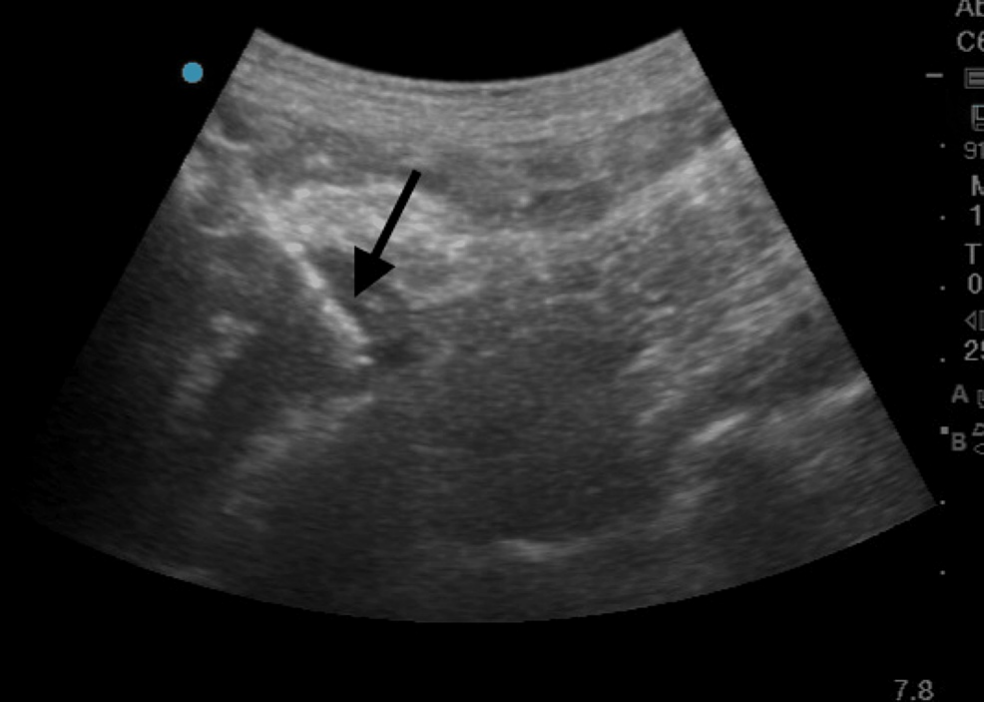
Ultrasonography-guided biopsy of mass lesion
